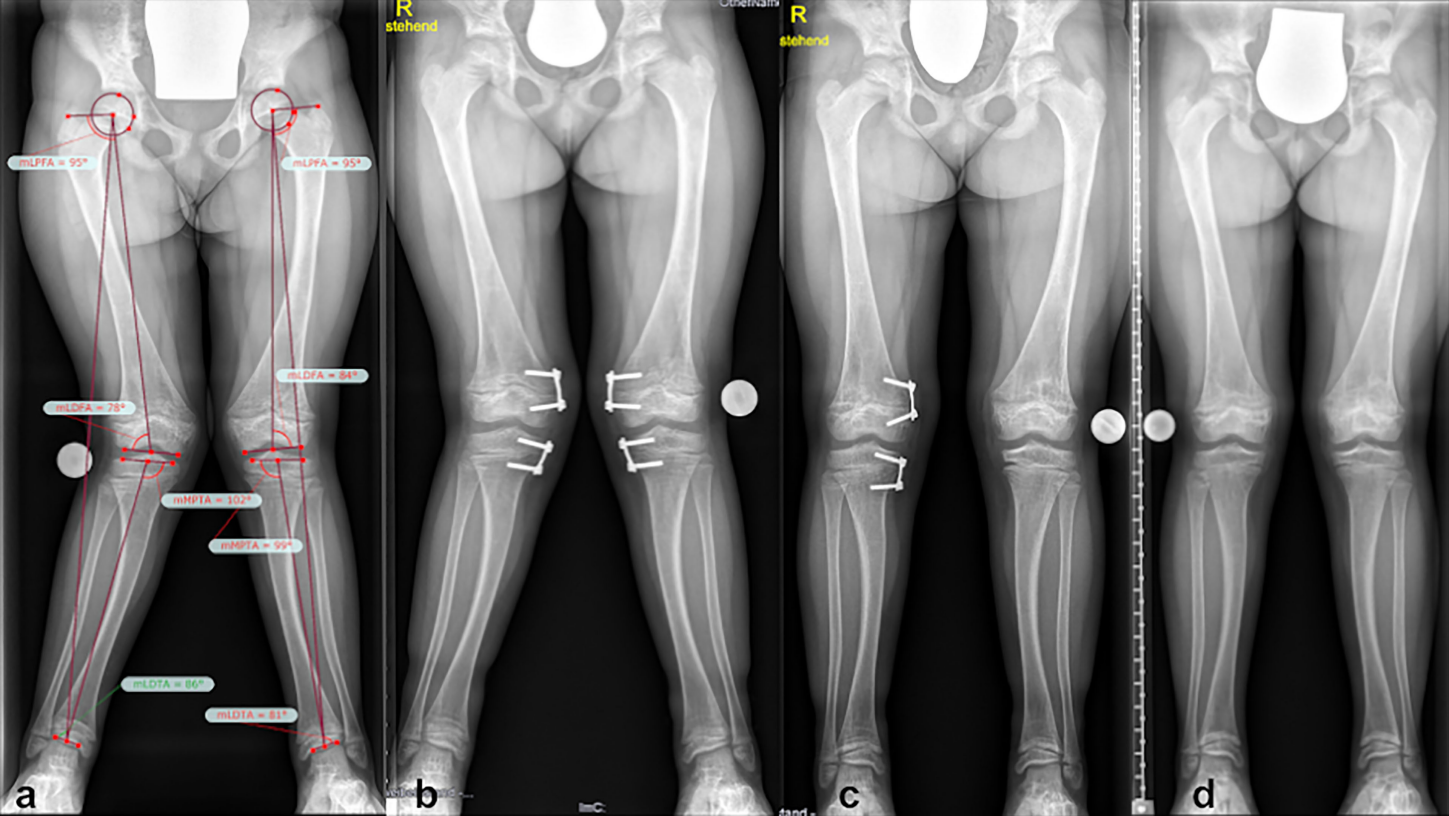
Lower limb deformity in a 7-year-old girl (case 3) undergoing Burosumab therapy. Full long leg standing radiographs and lower limb deformity analysis were obtained prior (A) to initiation, as well as during follow-up appointments. Valgus deformity was observed and a temporary hemiepiphysiodesis procedure performed in both legs (x-ray 2 months postoperative) despite persistent rachitic status (B). Normalization of MAD in one leg occurred sooner (C). As a sign of valgus deformity rebound, a mild recurrence of lateralization of the MAD after successful guided growth surgery despite Burosumab therapy was observed at the last follow-up 12 months after plate removal (D).
